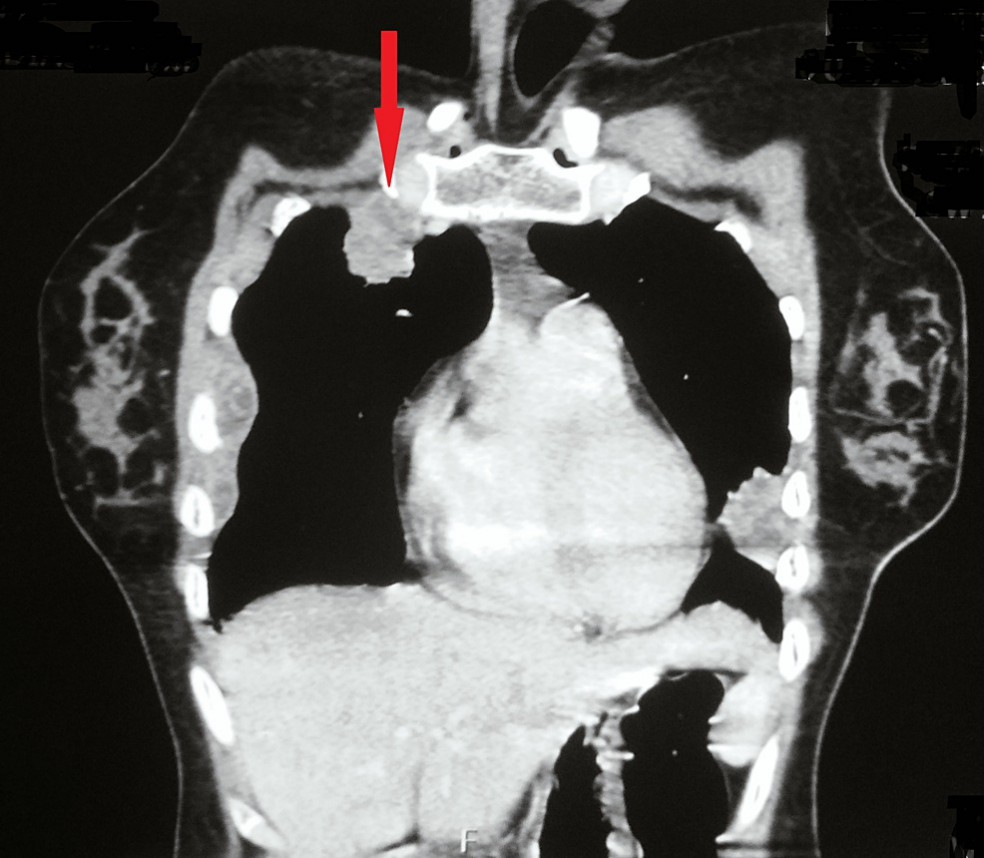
MDCT of the chest showing collection with moderately thick enhancing walls in the right pectoralis muscle MDCT - multidetector computed tomography
